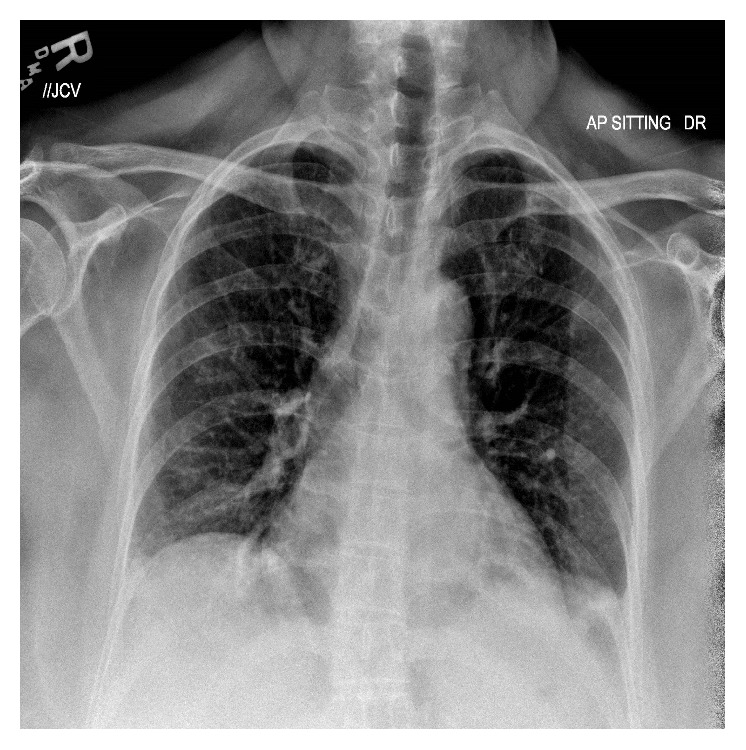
Chest X-ray showing hypoinflated lung fields, with basal atelectasis and elevated hemidiaphragms.
